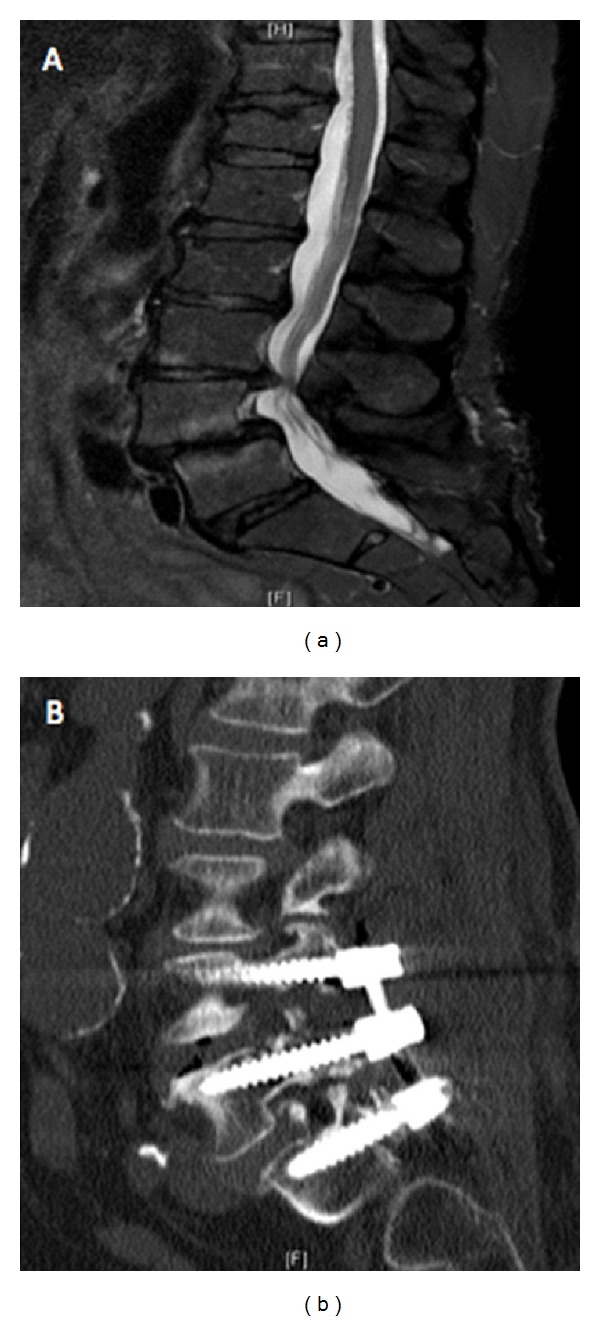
(a) Preoperative lateral MR image of a 66 y/o female with L4/L5 and L5/S1 spondylolisthesis and neuroforaminal stenosis; (b) Post-operative lateral MR image from a patient who underwent an MI-TLIF for spondylolisthesis at L4/L5, L5/S1.
